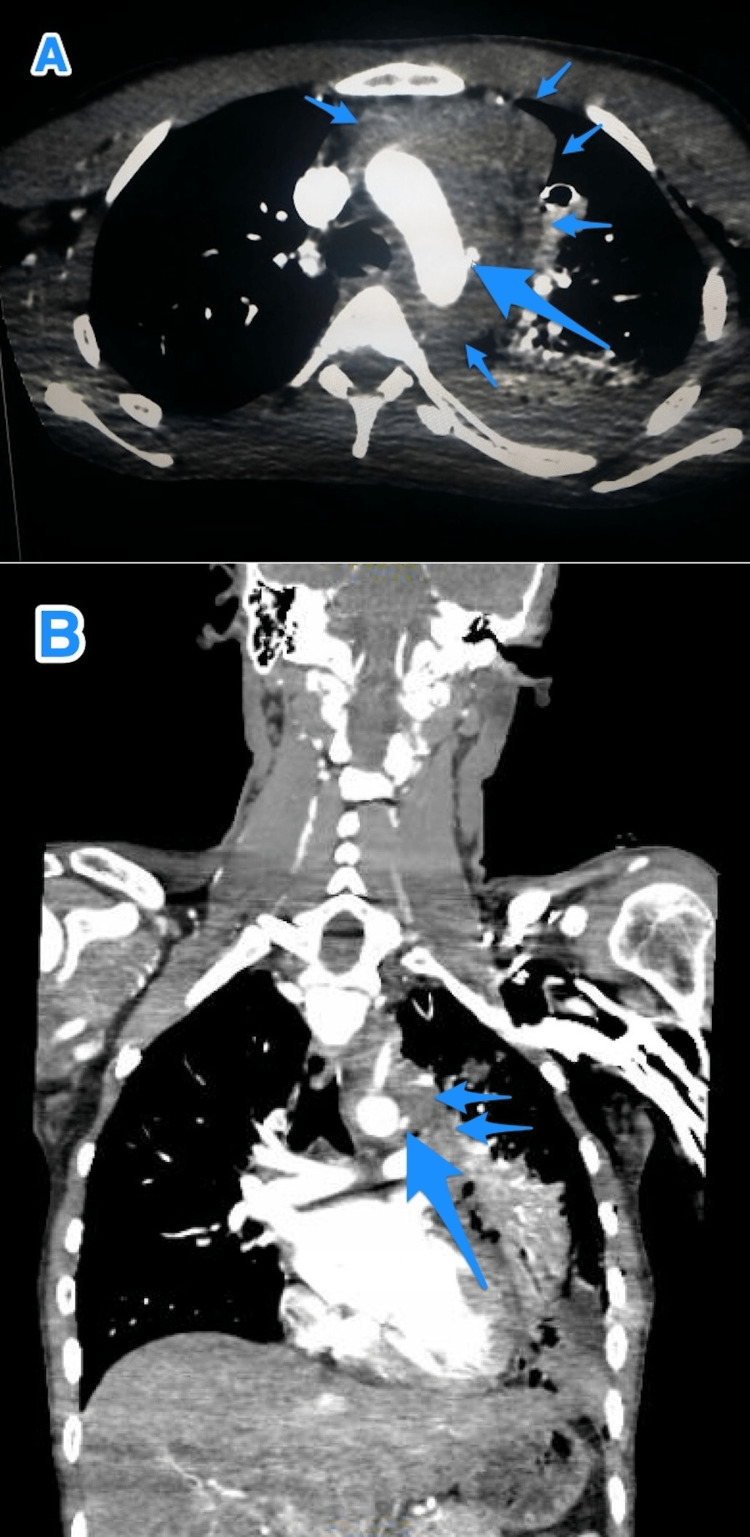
(A and B ) A chest CT angiography shows an aortic arch injury with extravasation of contrast (large arrow) about 1.5 cm from the left subclavian artery (zone 3) and surrounded by a large hematoma (small arrows).
